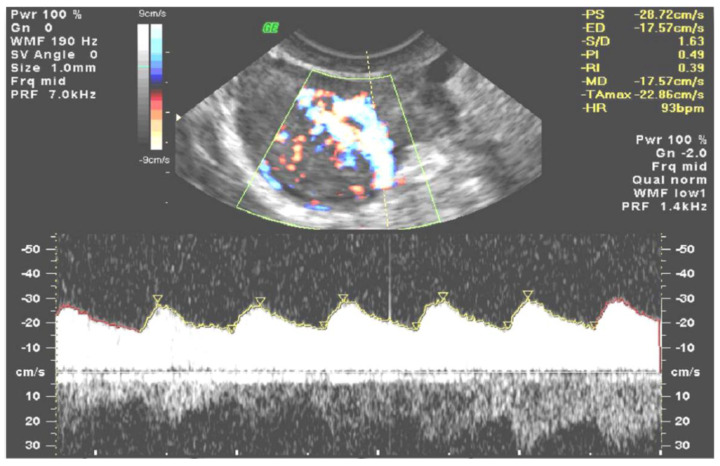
Spectral Doppler. Assessment of the blood flow in a single tumor vessel. The results of calculated 2D flow indices (S/D, PI, and RI) can be seen in the upper right corner.
